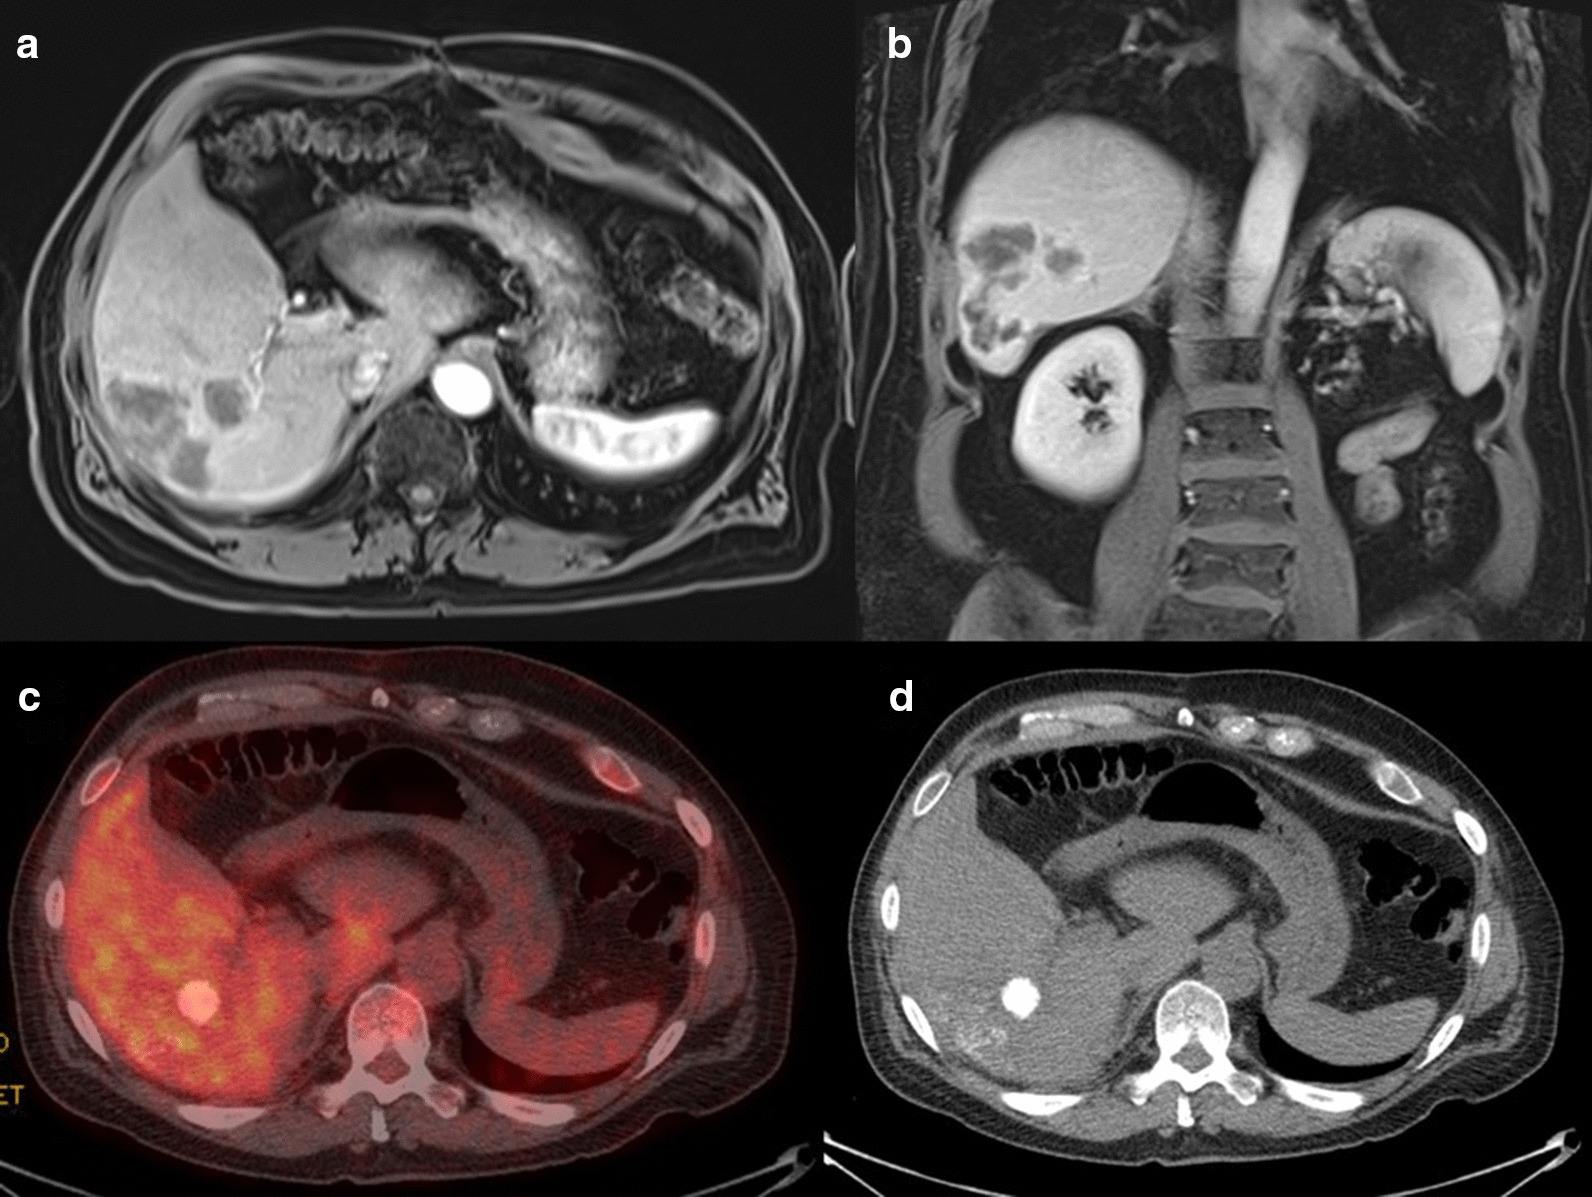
Post-radioembolization response. a, b 1-month post-radioembolization arterial phase post-Eovist T1-weighted fat-suppressed MRI shows decreased size of right hepatic lesions with no abnormal enhancement. c, d 3-month post-radioembolization PET/CT with [^18^F]FDG showed no residual activity in the right inferior hepatic tumor bed
